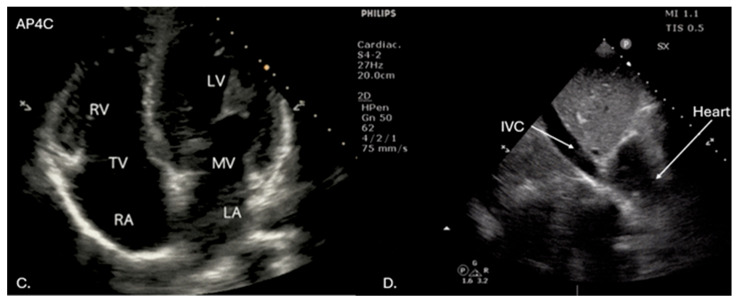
POCUS plays a major role in identifying reversible causes of cardiac arrest. Standard cardiac POCUS views are shown in (A–D) and include the parasternal long and short axis views (PLAX, PSAX), apical four chamber view (AP4C), subxyphoid view (SX), and inferior vena cava (IVC). Patients may be in shock, which is classified by its cause and includes cardiogenic, obstructive, and hypovolemic. The Sonography in Hypotension and Cardiac Arrest (SHoC) protocol is one example of a systematic process to evaluate potential causes of hypotension and arrest [4,5]. Using this protocol, POCUS users should evaluate the heart with SX and PLAX views, the IVC for increased diameter > 2 cm and reduced respiratory variation (although this is not reliable if the patient is on positive pressure ventilation), anterior and lateral chest views for edema or effusions, the aorta for aneurysm or dissection, the deep veins for thrombus, and the abdomen/pelvis for hemorrhage [4,5]. Challenges of using POCUS during cardiac arrest include its operator dependence, potential for misinterpretation (e.g., distinguishing true cardiac standstill from minimal activity), and the risk of prolonging pulse checks. The Cardiac Arrest Sonographic Assessment (CASA) protocol is an example of a systematic approach to POCUS in cardiac arrest that can help mitigate these challenges [6,7]. Clattenburg et al. found that protocol implementation in 267 cardiac arrest patients over 19 months significantly reduced the duration of cardiopulmonary resuscitation (CPR) interruptions while ultrasound was being performed. Using the CASA protocol, pulse checks with POCUS were 4.0 s shorter (95% CI 1.7–6.3 post-intervention), and POCUS use increased from 64% pre- to 80% post-intervention [6,7]. Additional recommendations for safely incorporating POCUS into cardiac arrest algorithms include having a dedicated person whose only role is to perform the ultrasound and selecting the most experienced POCUS operator to perform the ultrasound. Finally, positioning the ultrasound probe on the chest in preparation for the pulse check and recording the video cine loop then stepping away from the patient for video review can also mitigate pulse check interruptions [6,7].
